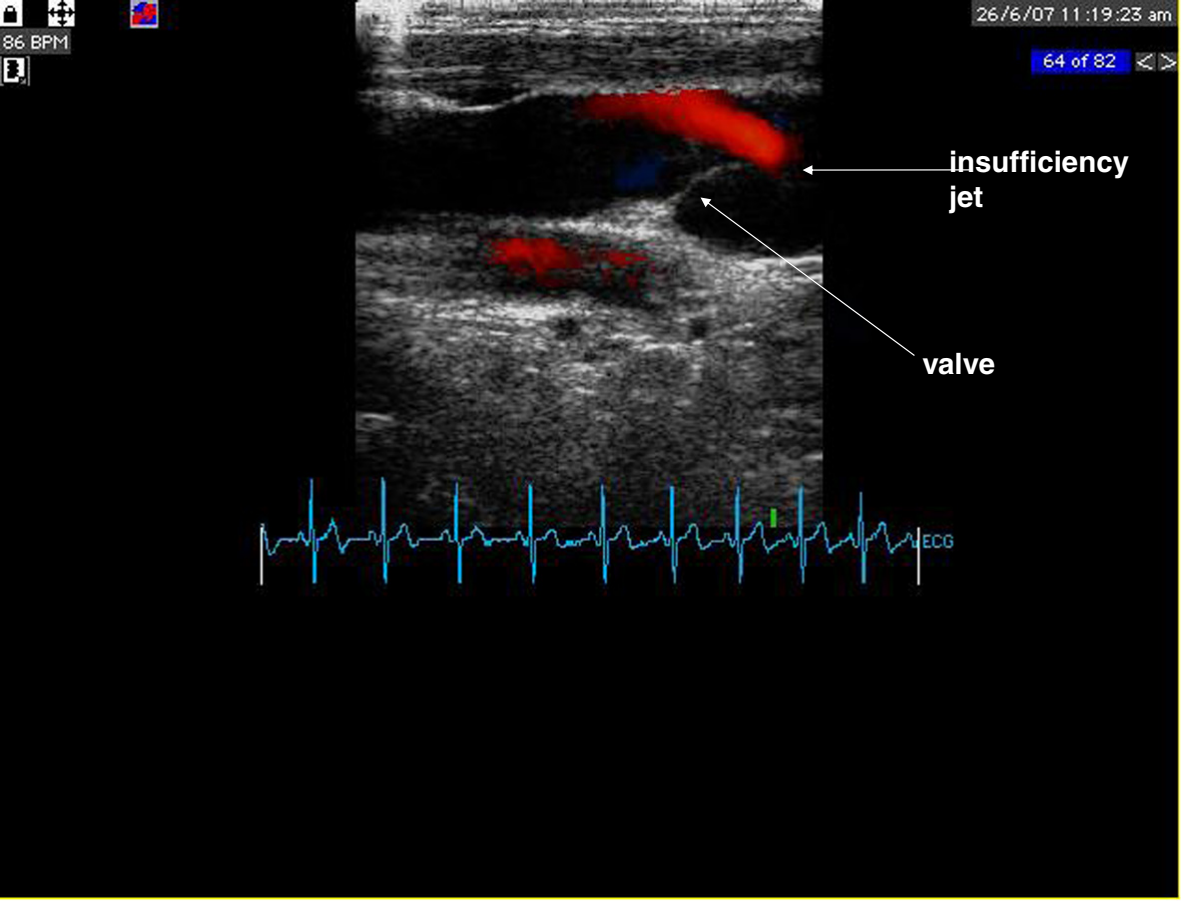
Ultrasound evaluation of internal jugular valve incompetence in colour Doppler mode. Insufficiency jet originating from the valve (red colour) is indicated by an arrow.
